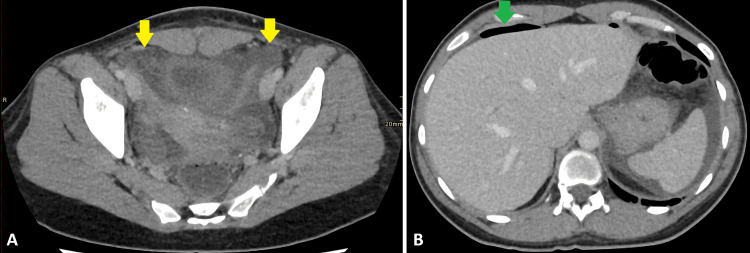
CT scan abdomen with contrast (axial views) Contrast-enhanced CT abdomen, axial views (A and B), showing expected postoperative changes in the form of free fluid (yellow arrows in A) and pneumoperitoneum (green arrow in B). CT: computed tomography
